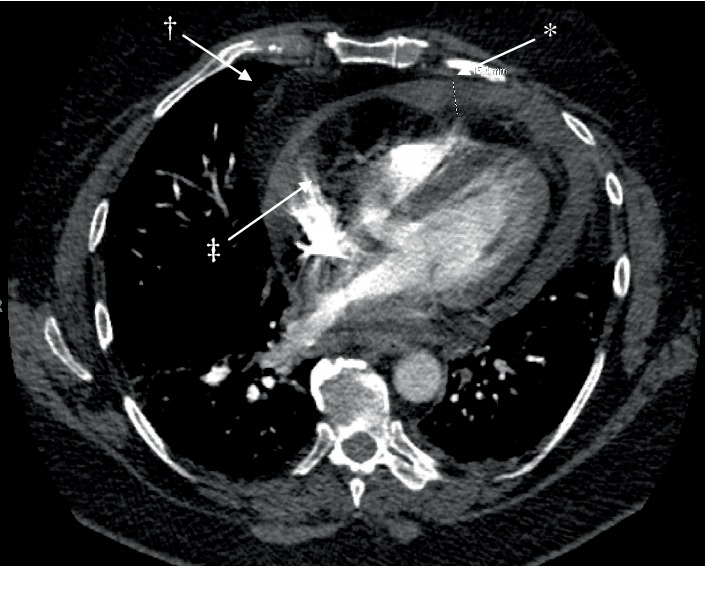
CT pulmonary angiography. Findings: ^∗^pericardial effusion: 15.4 mm (shown). ^†^pericardial mass; ^‡^epicardial fat.
